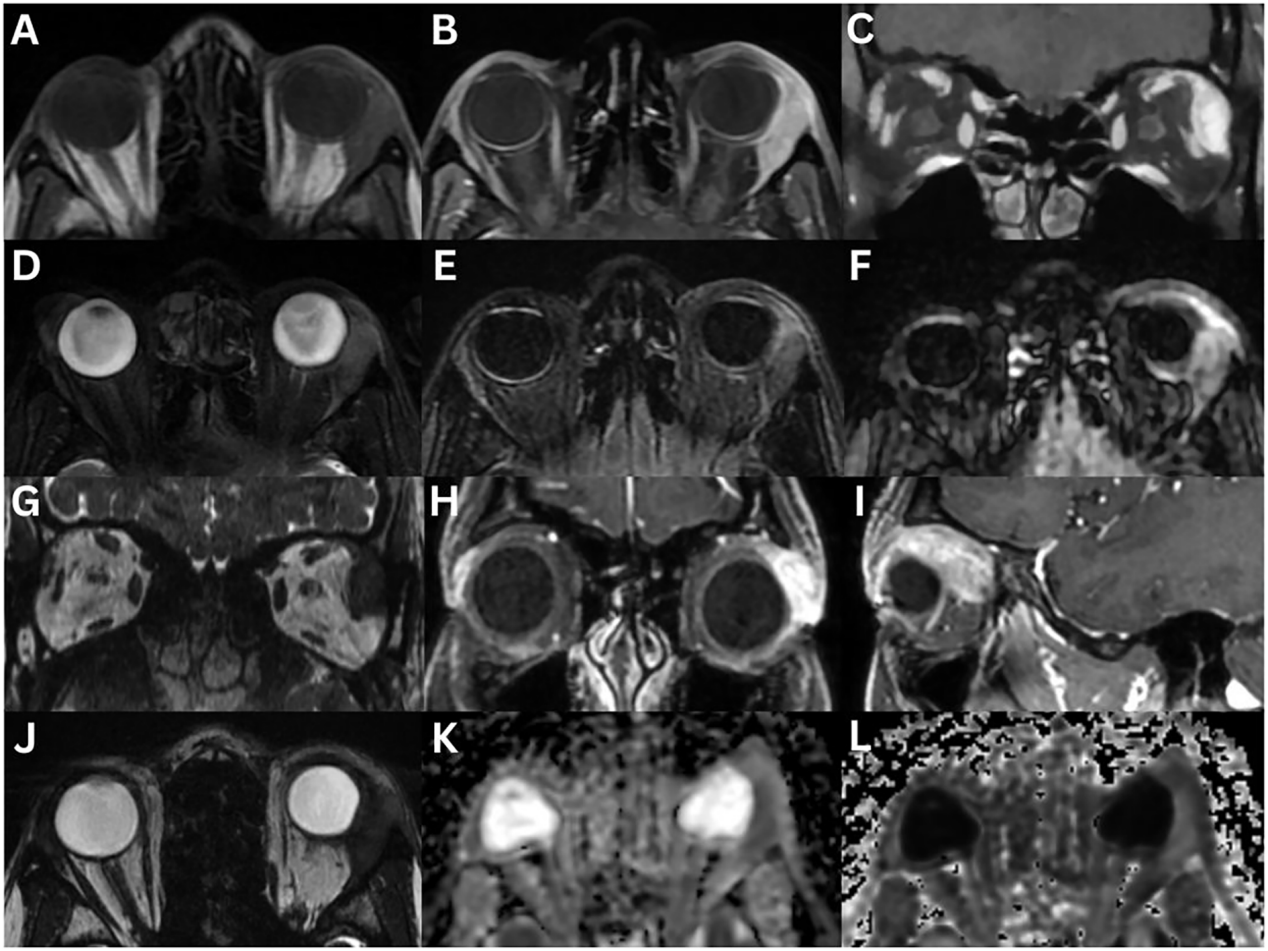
Idiopathic orbital inflammation of the left orbit. MRI T1-weighted axial (A), axial fat-suppressed contrast-enhanced (B), and coronal fat-suppressed contrast-enhanced (C), T2-weighted axial (D), axial fat-suppressed FLAIR (E), axial 3D DIR CUBE (F), coronal FIESTA (G), coronal contrast-enhanced (H), and sagittal contrast-enhanced (I) demonstrates enlargement of the left lacrimal gland. The lesion is homogeneous and solid, with intermediate signal intensity on T1- and T2-weighted images, and demonstrates marked contrast enhancement. Axial 3D Fiesta-C is also shown (J). No restricted diffusion was observed on axial ADC (K) or eADC (L).
